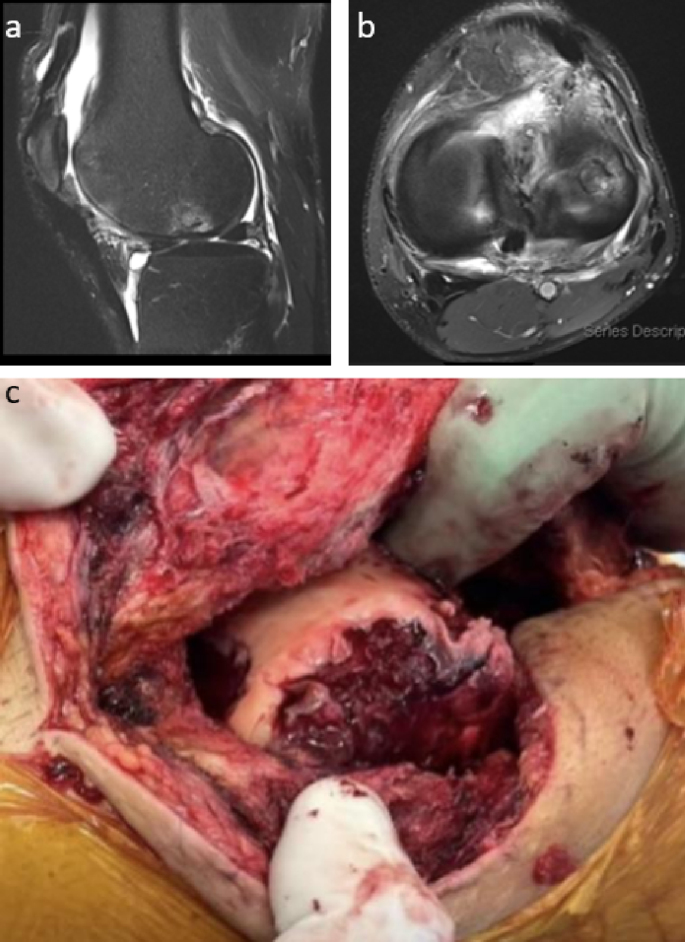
Gross intraoperative appearance and MRI findings during initial presentation. Both (a) sagittal and (b) axial MRI imaging of the left knee revealed moderate-sized joint effusion with enhancement and thickening of the synovial lining, as well as foci of cortical irregularity and bone marrow edema suggesting septic arthritis. (c) Initial surgical washout of the knee yielded extensive exudate following debridement of the joint capsule and synovium.
